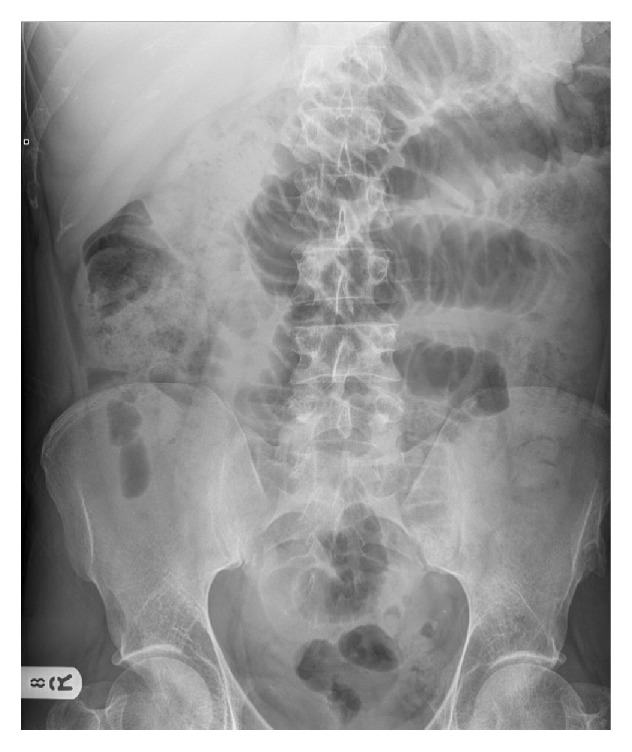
Abdominal plain film radiography demonstrating multiple dilated small bowel loops.
